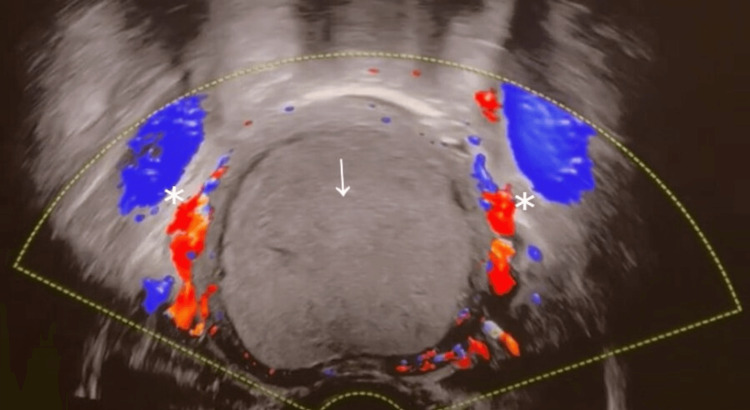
Hematometra on ultrasound scan (arrow) Hematometra seen as homogeneous fluid collection on the ultrasound scan (marked by an arrow). In addition, you can clearly note the normal blood supply around the uterus which has no continuation with the hematometra itself (both stars).
